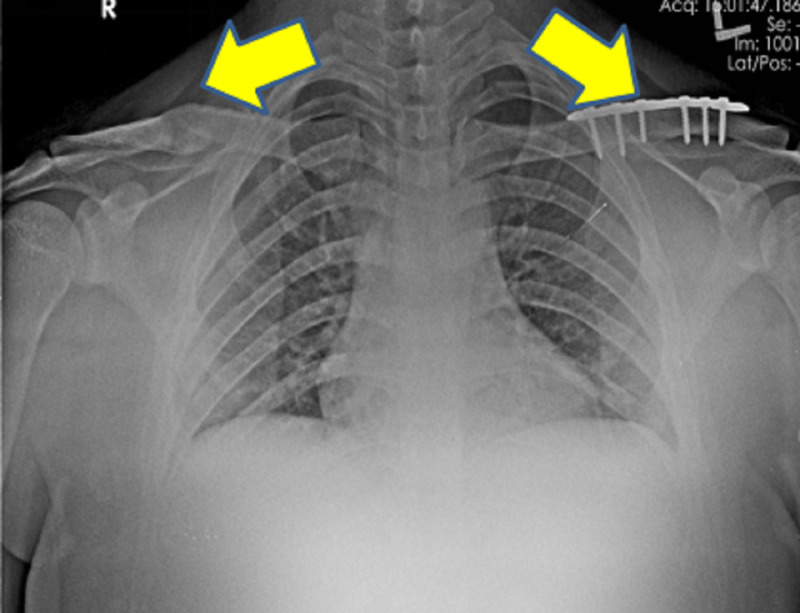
The plain radiograph of the chest with bilateral shoulder anteroposterior view at six months follow up showing locking compression plate in position for left clavicle fracture and united right clavicle fracture
